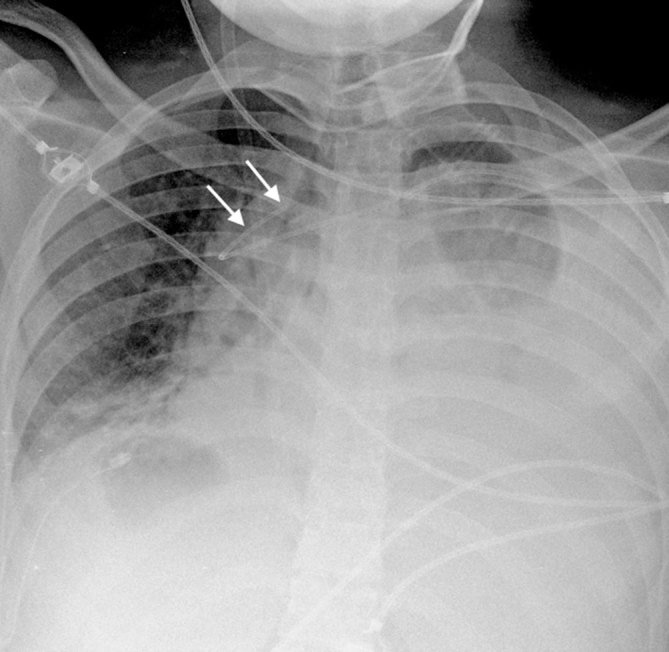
Frontal chest radiograph 10 days after PICC insertion shows large bilateral pleural effusions with atelectasis. The PICC tip remains curved (white arrows). PICC. peripherally inserted central venous catheter.
